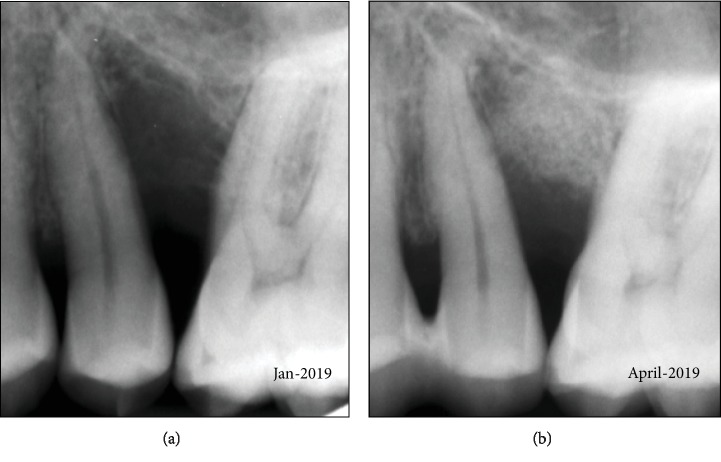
Radiographs before (a) and after surgery (b).
